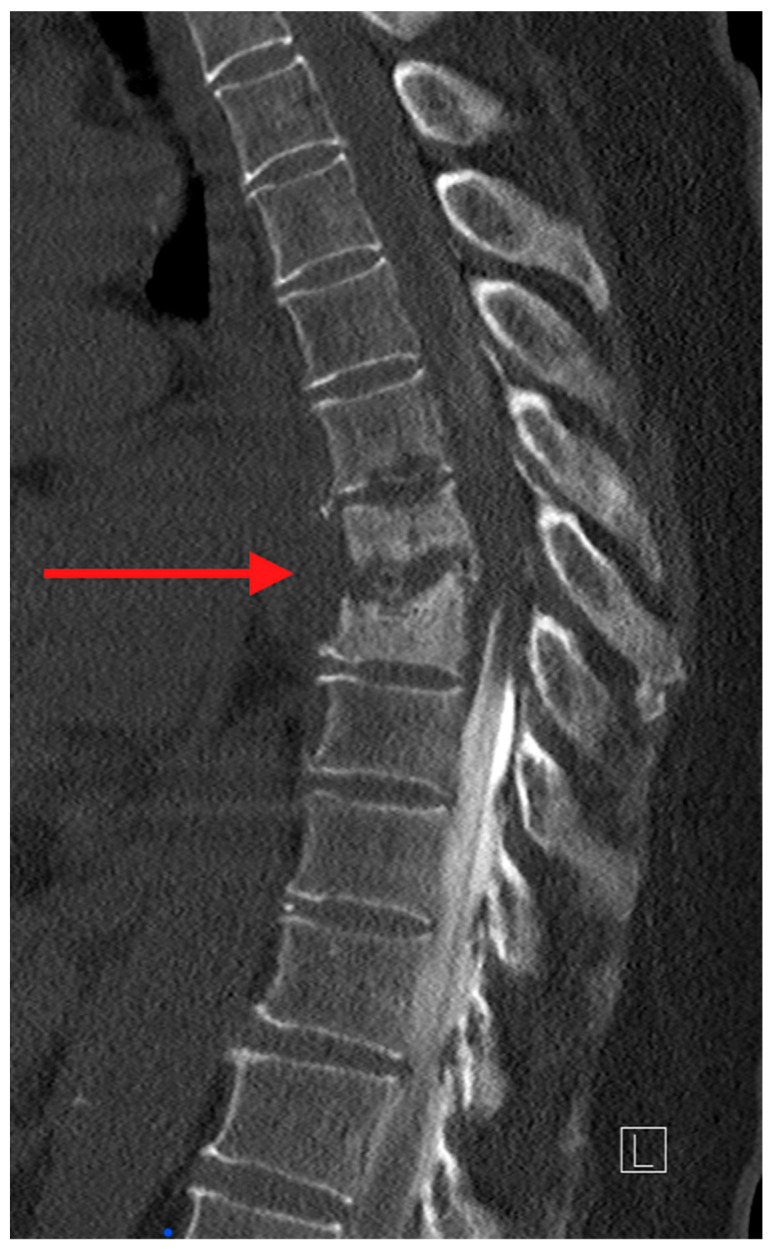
Sagittal CT myelogram of the thoracic spine. The red arrow is pointing to the destructive changes of several vertebral bodies in the mid-thoracic spine. Also noted is the myelogram dye in the spinal canal. It was injected caudal to cranial and the abrupt disappearance of the column of dye at the infected level suggests high-grade cord compression [4].
